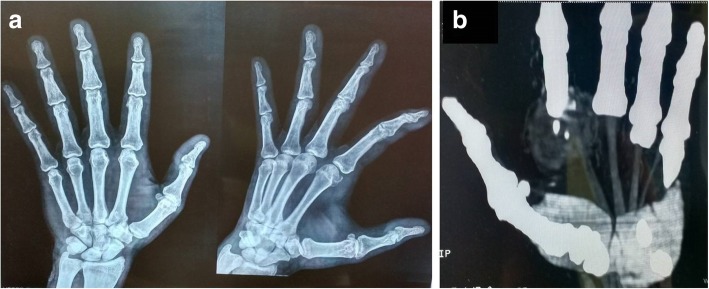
a Anteroposterior and lateral X-ray films of left hand showing a soft tissue lesion. b Contrast-enhanced computed tomography of left hand showing a soft tissue mass lesion, with peripheral enhancement and central necrotic areas in radial palmar soft tissue overlying second metacarpophalangeal region with no obvious bony osteolysis
